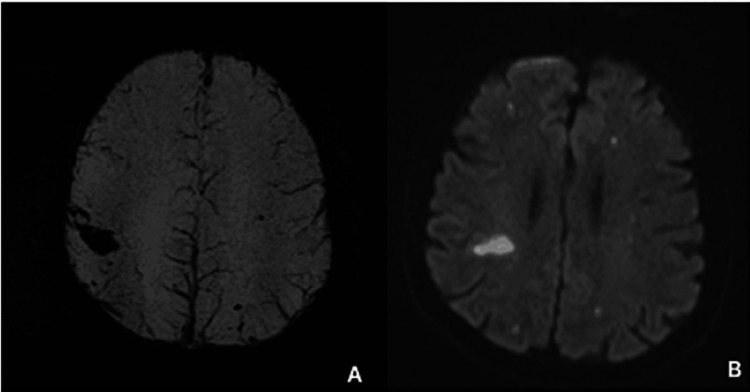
Brain MRI scan Brain MRI showing blooming foci in susceptibility weighted imaging (SWI) (A) corresponding to regions with restriction in the diffusion study (B) and assumed as ischemic injuries with discrete foci of hemorrhagic transformation. Multiplicity and distribution suggesting an embolic ethology.
